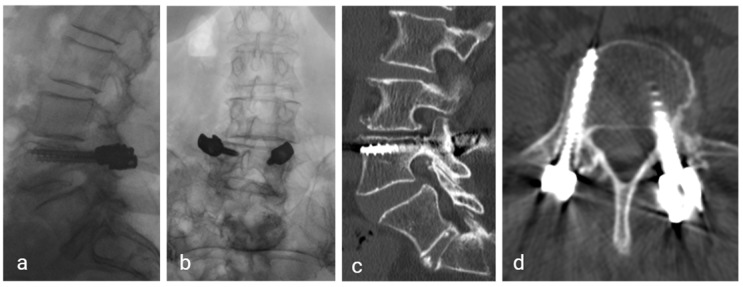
Postoperative follow-up at 6 months, demonstrating stable grade 1 spondylolisthesis and fracture consolidation. (a) Sagittal and (b) axial radiograph. (c) Sagittal and (d) axial CT showing the bicortical trajectory of the pedicle screws.
